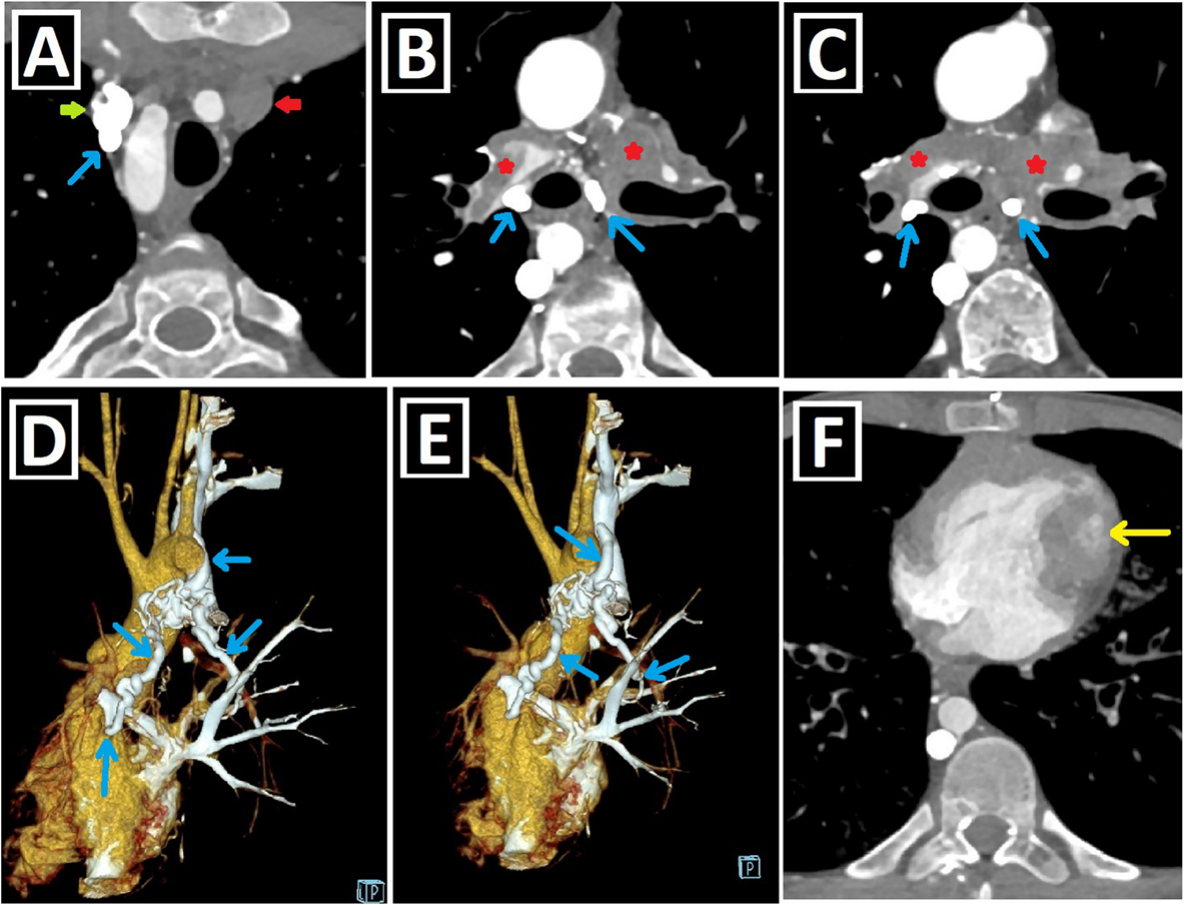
Levoatriocardinal vein-2. Axial (a, b, c, f) and 3D VRT (d, e) CT images of a patient with double SVC (green and red arrows, a) and complex cardiac anomaly, who underwent bicaval Glenn shunt operation. Axial CT images (b, c) depict the thrombus extending from the right Glenn shunt to confluent pulmonary arteries (red stars). Due to the thrombus in the right Glenn shunt, the distribution of the contrast agent injected from the right arm into the mediastinal collaterals and the azygos system is observed. Axial (b, c) and 3D VRT reconstructed (d, e) CT images indicate bilateral vascular structures, which are compatible with LACV (blue arrows), originating from right Glenn shunt, coursing in the posterior of bilateral pulmonary arteries and draining into the right and left upper pulmonary veins. Please note that LACV may accompany LOLs (yellow arrow shows hypoplastic left heart, f), may be seen together with PLSVC, may be associated with any venous structure in the cardinal system not only left brachiocephalic vein, and in some cases, may have blood flow in the craniocaudal direction
